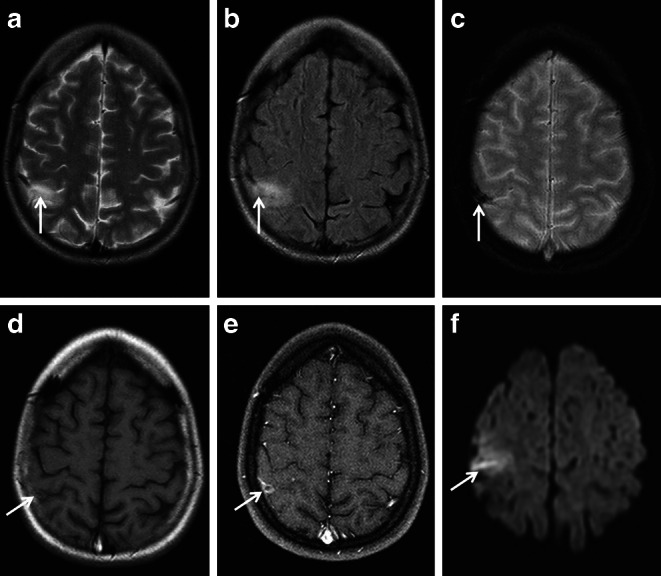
Cortical vein thrombosis as a possible mimic of cortical superficial siderosis (cSS) in a 27-year-old woman with right-sided headache and sensory Jacksonian seizures. MRI demonstrating cortical hyperintense lesion postcentral parietal right (a: T2 WI ax.; b fluid attenuated inversion recovery [FLAIR] images ax.; *arrow*), signal loss and “blooming” of the central vein (c, T2*WI ax.; *arrow*) without cSS, circumscribed peripheral contrast enhancement (d,e T1 WI ax., post contrast T1 WI ax.; *arrow*) and restricted diffusion of the thrombus (f, diffusion-weighted imaging [DWI] ax., b = 1000 s/mm^2^, *arrow*)
